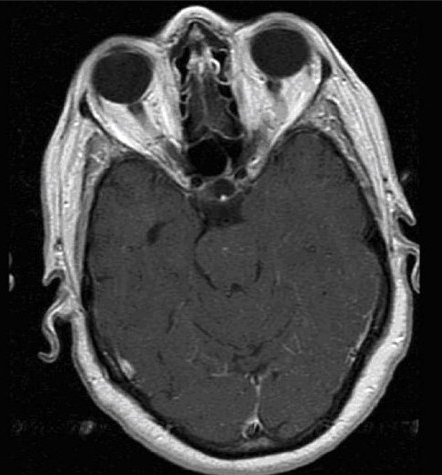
(a)T1-weighted MR images with gadolinium. Left temporal lobe tumor shows no enhancement but mild mass effect on the left ambient cistern
